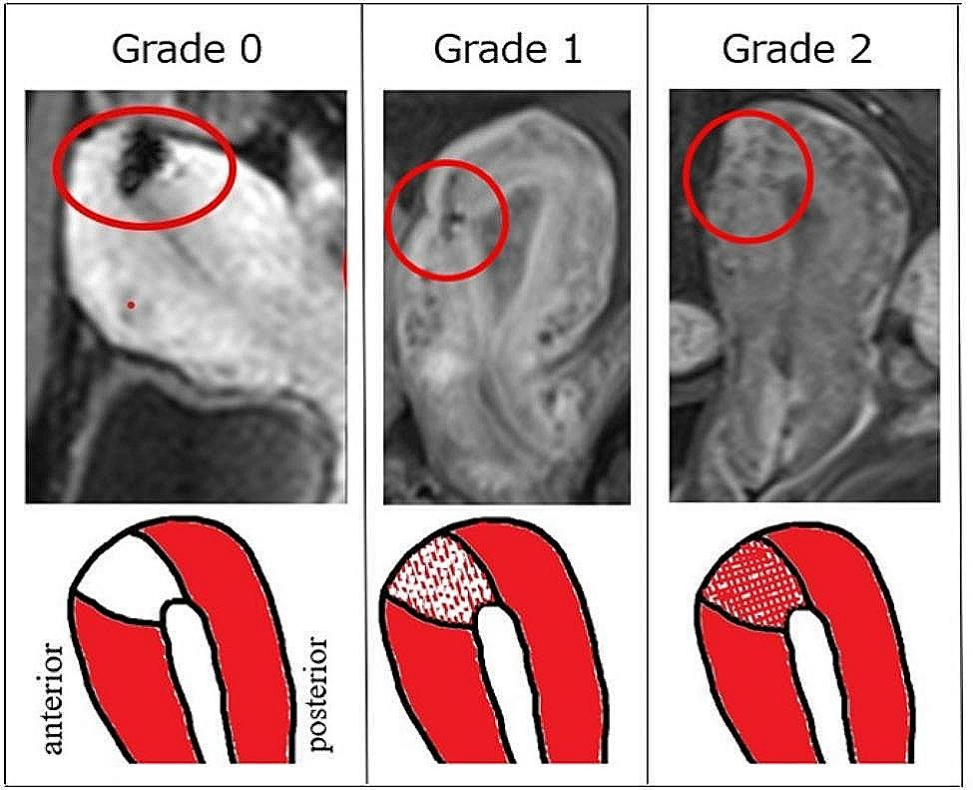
MRI evaluation of vascularization in scar 12 months after TUFI using dynamic contrast-enhanced images The percentage of enhanced area in the scar was visually evaluated and classified into three categories as follows: Grade 0: the scar area was not enhanced Grade 1: parts of the scar area were enhanced Grade 2: ≥80% of the scar area was enhanced
